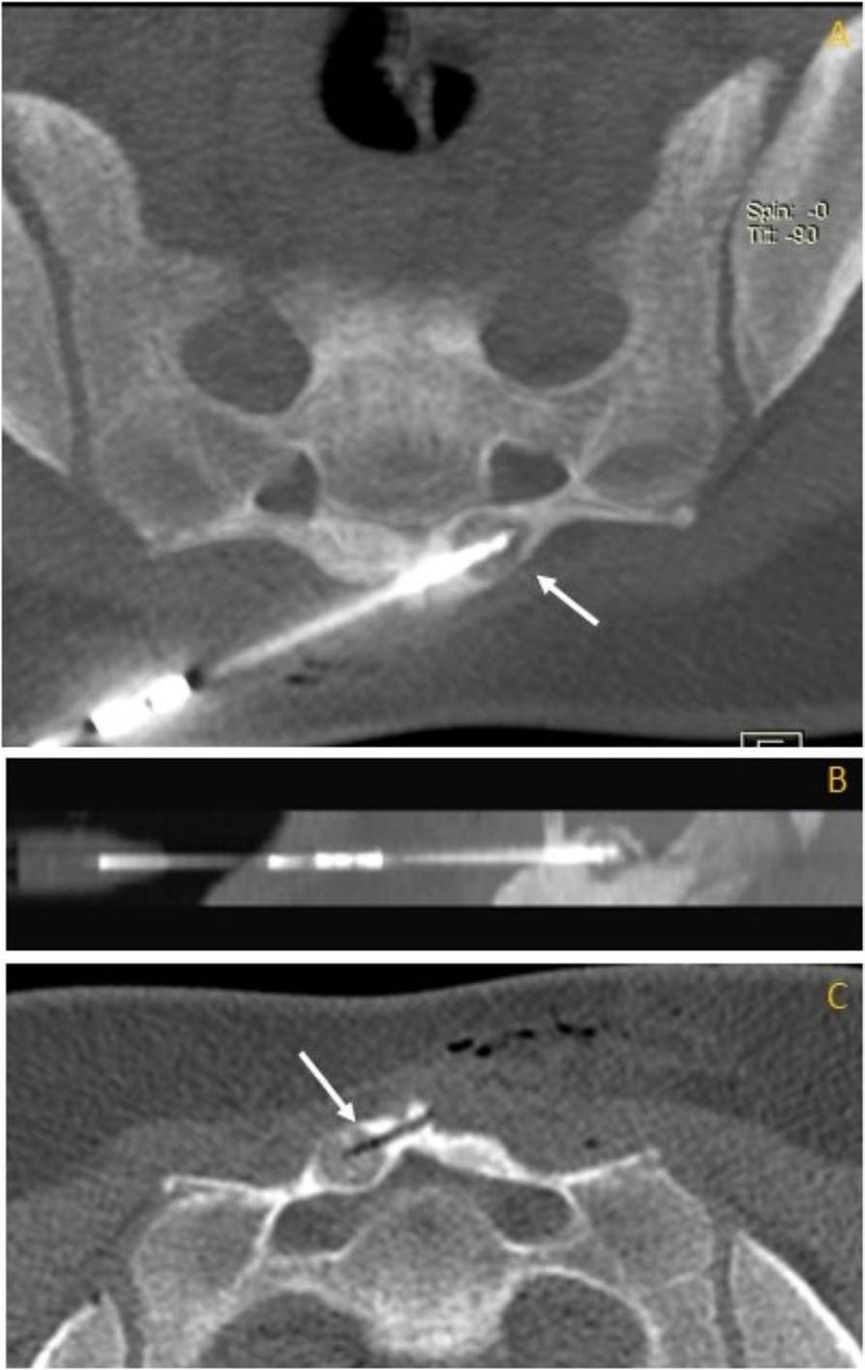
Radiofrequency ablation of an osteoid osteoma of the sacrum in a 16-year-old female patient. High-resolution CT of the sacrum in the bone window in axial (A) and sagittal (B) image during radiofrequency ablation depicts the intralesional placement of the needle tip inside the oval-shaped intracortical lying geographical osteolysis measuring CC 10 × LL 12 mm with pronounced perifocal sclerosis and no periosteal reaction, corresponding to osteoid osteoma (arrow). C Postprocedural bone defect and gas in the soft tissues on axial CT image after RFA (arrow). After 12 years of follow-up, the patient is recurrence-free
